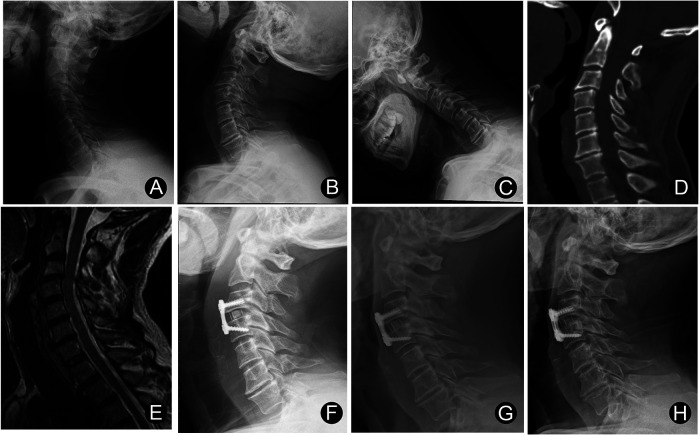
Male, 57 years old: C3/4 ACDF was experienced. (A–C) Cervical spine x-ray showing C3/4 instability. (D) Preoperative cervical CT sagittal image. (E) Magnetic resonance imaging (MRI) showing no significant compression of the spinal cord. (F) x-ray of cervical spine 3 months after surgery. (G) x-ray of cervical spine 12 months after surgery. (H) x-ray of cervical spine 24 months after surgery.
